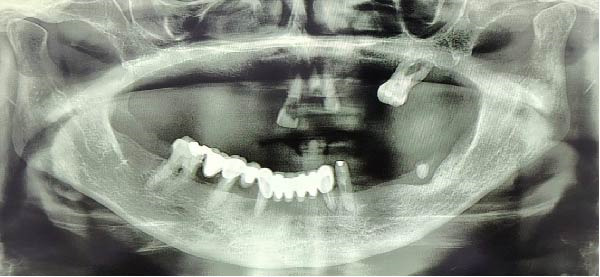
Orthopantomography revealed atrophic maxilla.
